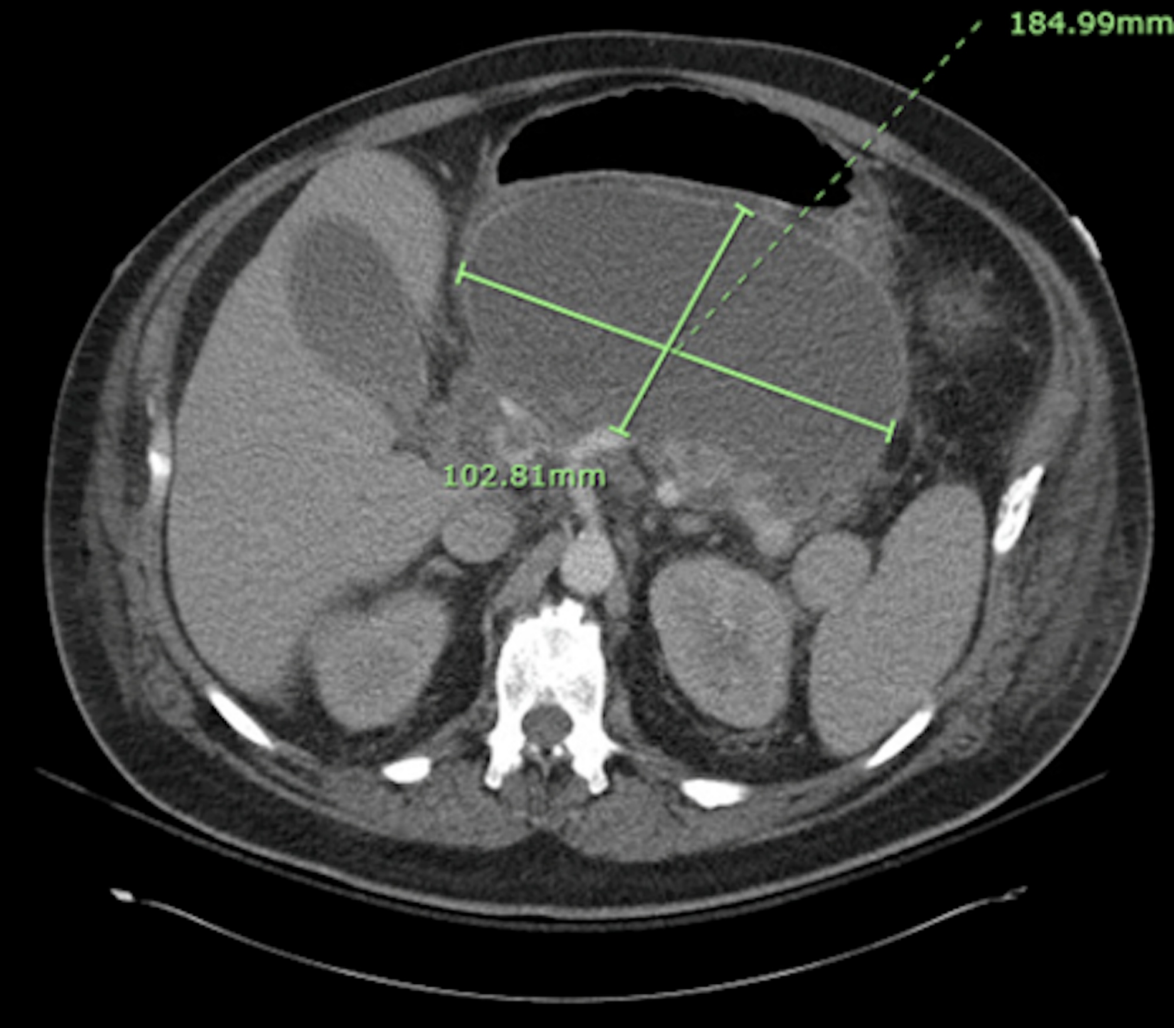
CT of the abdomen and pelvis showing an 18.5 x 10.3 cm peripancreatic cyst containing debris, most consistent with a pancreatic pseudocyst.
